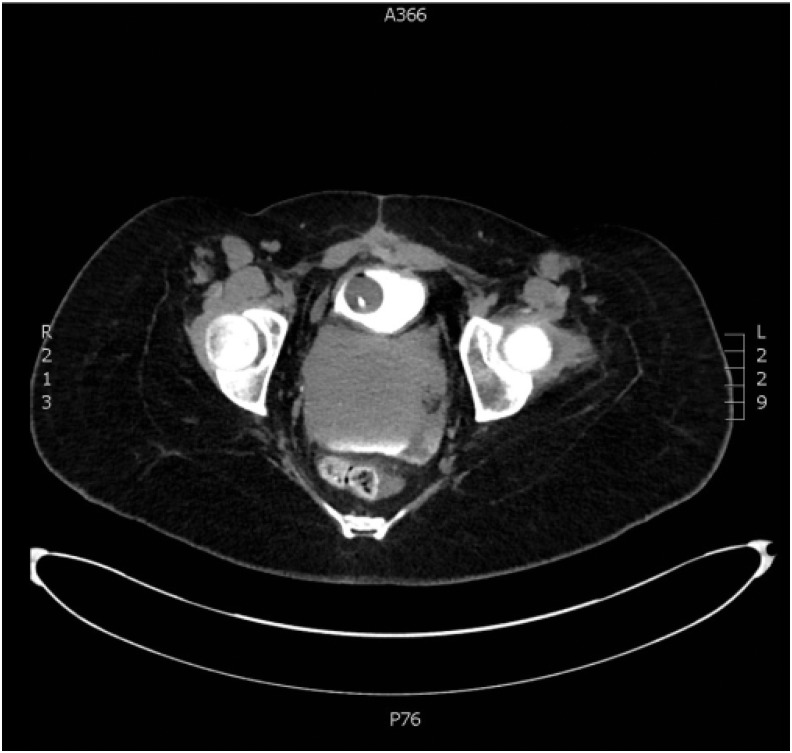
Axial imaging of CT cystogram showing posterior fluid collection.
